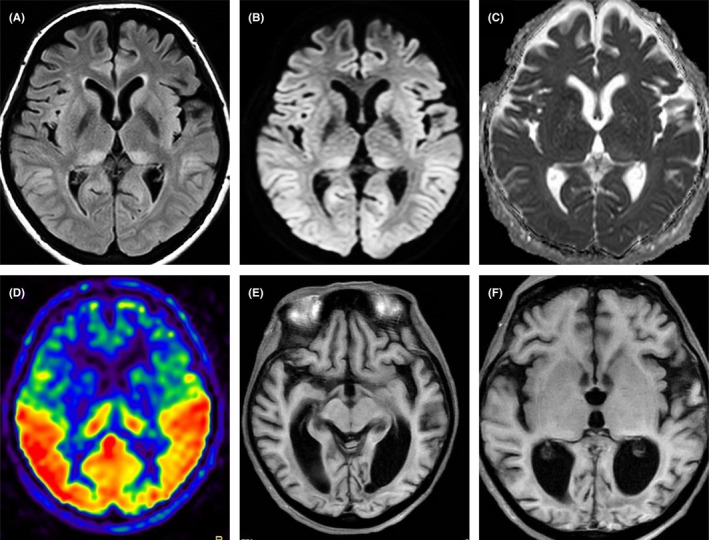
Head MRI of Case 2. MRI on admission shows high‐intensity lesions in the occipital lobe and bilateral thalamus in FLAIR and DWI (A, B). These lesions show isointense in ADC (C). ASL shows hyper‐perfusion in the occipital and thalamic lesions (D). One‐year follow‐up MRI shows occipital lobe‐dominant brain atrophy (E, F)
